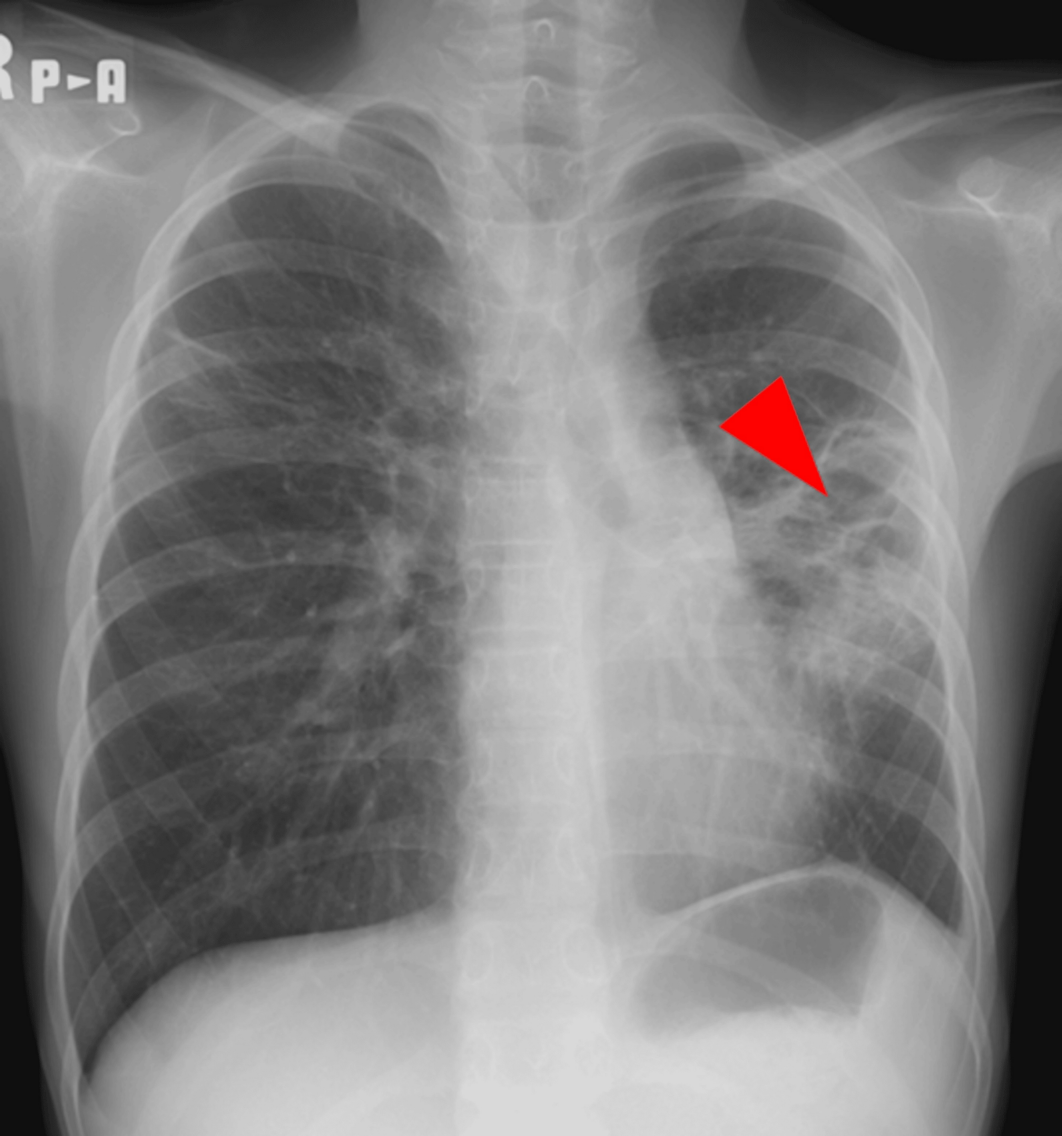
Chest X-ray at hospital discharge. Chest X-ray showed improved consolidation in the left lobe (arrowhead).
